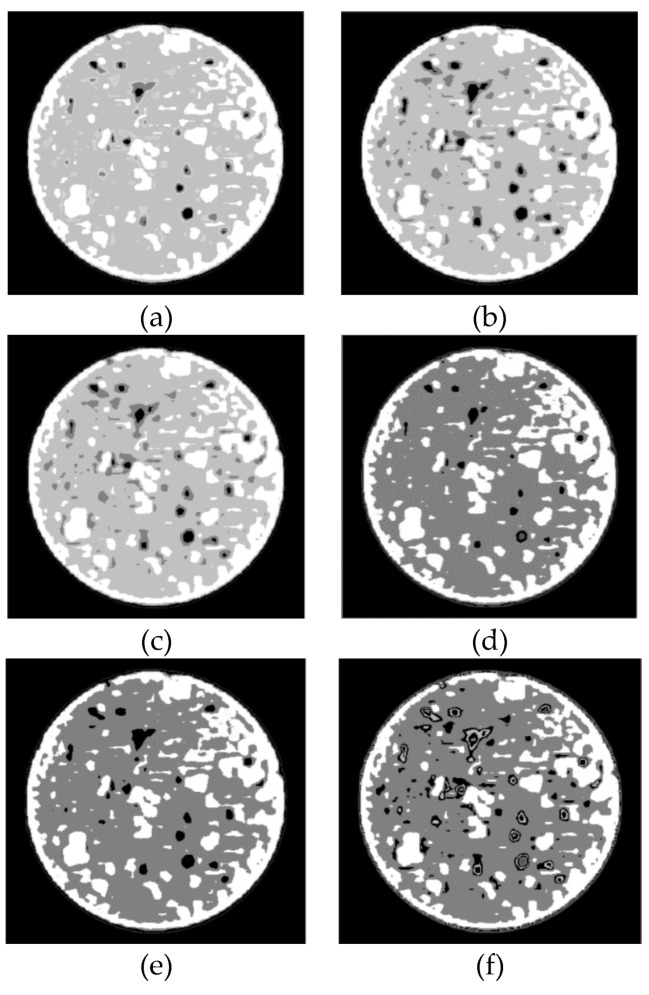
Grayscale images obtained by CT scanning. (a) Freeze-thaw = 0; (b) Freeze-thaw = 1; (c) Freeze-thaw = 3; (d) Freeze-thaw = 6; (e) Freeze-thaw = 10; (f) Freeze-thaw = 15.
